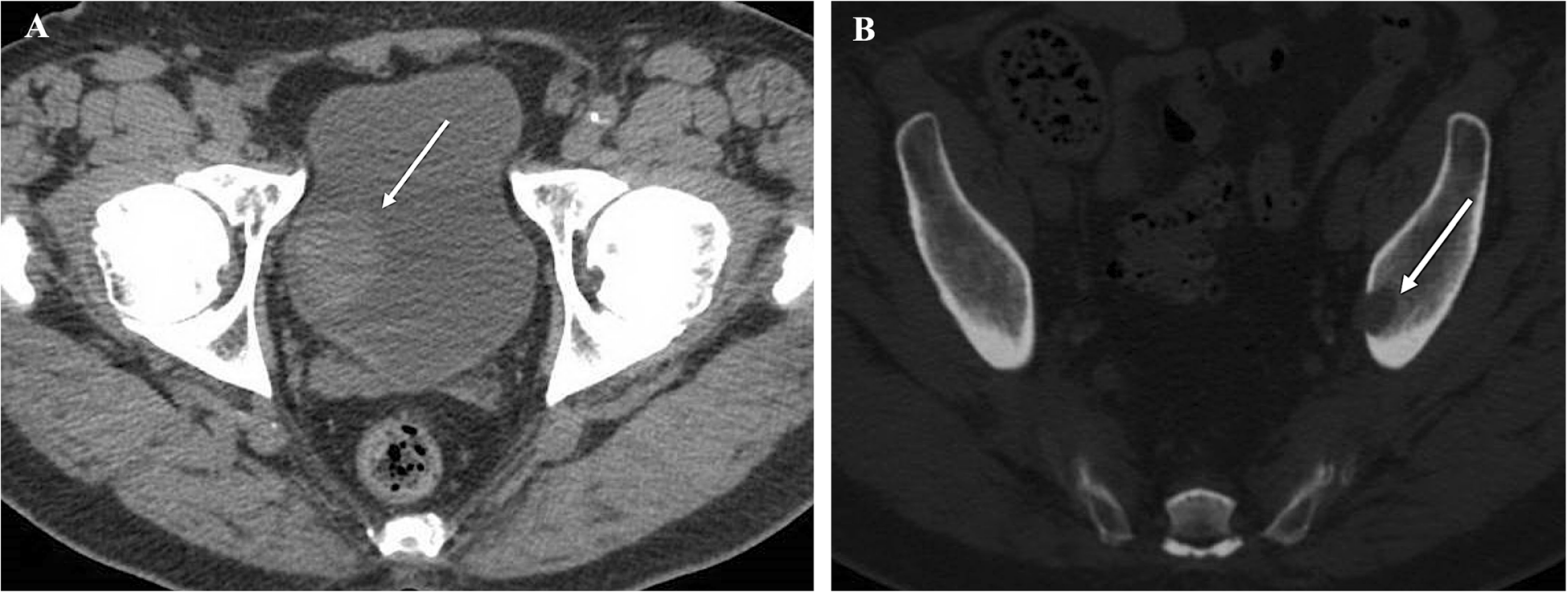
a Non-contrast CT abdomen and pelvis demonstrates a hyperattenuating intraluminal bladder mass (white arrow). b Non-contrast CT abdomen and pelvis reveal a lytic lesion with cortical destruction and extraosseous soft tissue extension in the left ilium (white arrow), consistent with osseous metastasis
